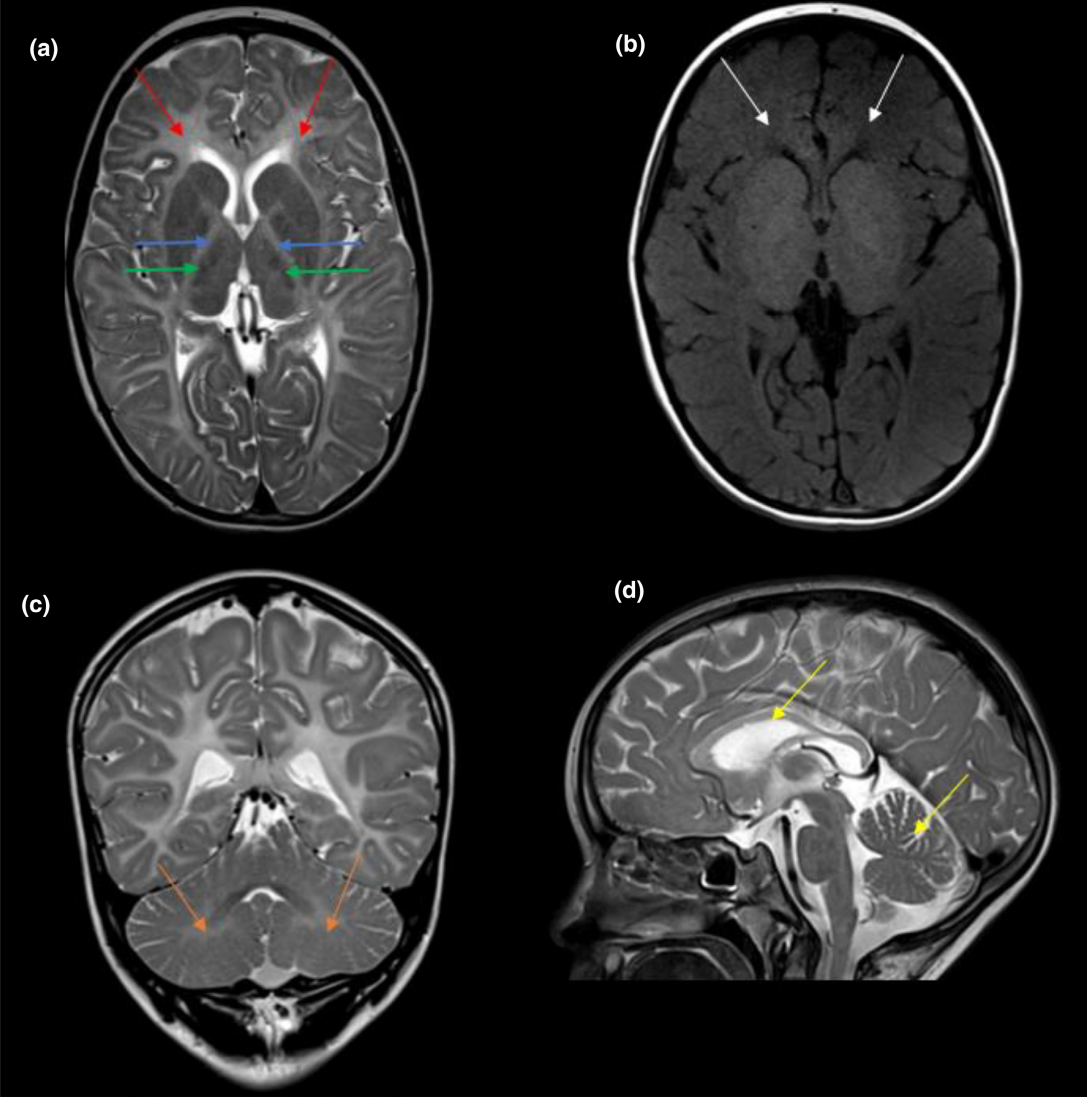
Brain MRI imaging of a five‐year‐old patient in our series (Patient 2). Axial T2‐weighted image (a) showing diffuse white‐matter hyperintensity with U‐fiber involvement (red arrows) and posterior limb of internal capsules involvement (blue arrows). A relative T2 hypointensity of the ventrolateral nuclei of the thalami can also be noted (green arrows). Axial T1‐weighted image (b) showing corresponding T1 isointensity in comparison with the cortical gray matter, compatible with a hypomyelinating process (white arrows). Coronal T2‐weighted image (c) showing diffuse hypomyelination including cerebellar white matter (orange arrows). Sagittal T2‐weighted image (d) showing moderate cerebellar and corpus callosum atrophy (yellow arrow). Also note hypomyelination involvement of the corpus callosum.
